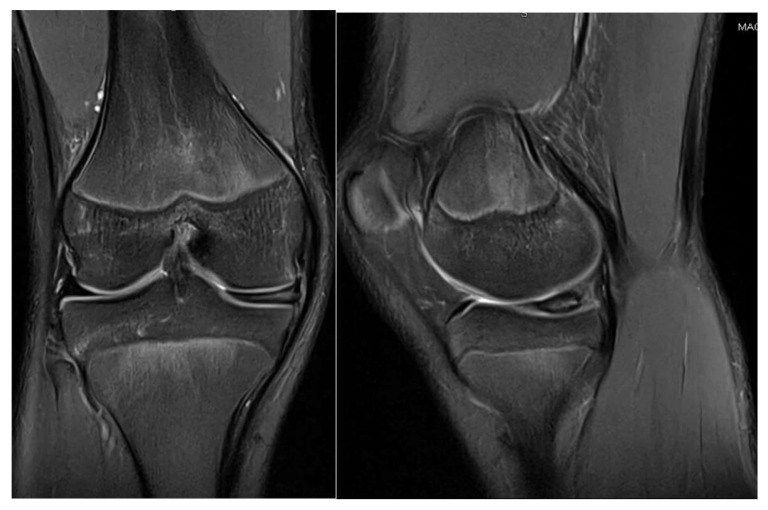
Patient G.L. (14 years old). Preoperative MRI of the right knee with symptomatic medial discoid meniscus (left—coronal T2 view; right—sagittal T2 view). The coronal view demonstrated a widened and abnormally thickened medial meniscus extending into the intercondylar notch, characteristic of a discoid morphology. The sagittal view showed a horizontal signal cleft in the posterior horn, consistent with a horizontal tear. These findings led to an arthroscopic partial meniscectomy and meniscal suturing.
